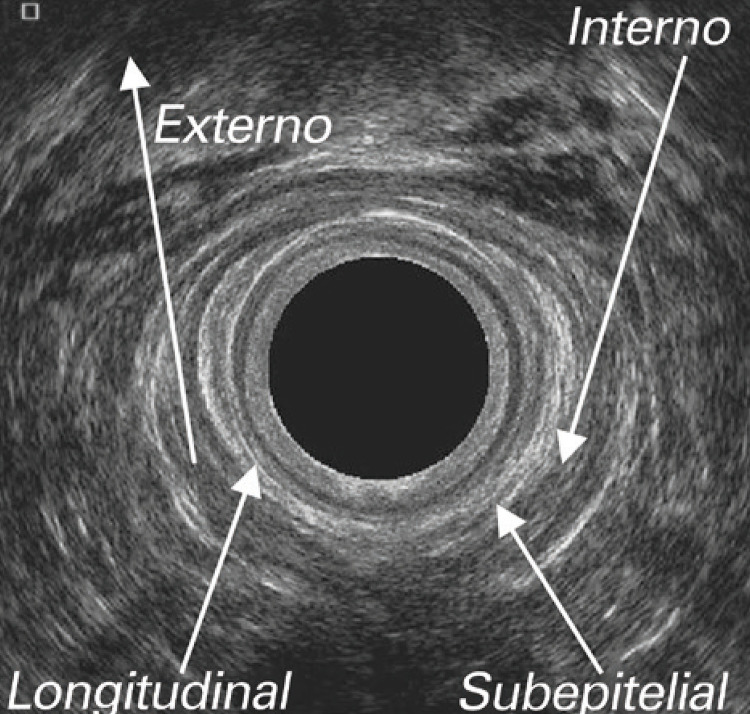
Anatomia normal do canal anal como vista na endossonografia anal4.3 Radiografia4.3.1 Modalidades atuais em uso clínico rotineiro4.3.1.1 Urografia intravenosa: fornece descrição anatômica do trato urinário superior, dos ureteres e da bexiga, bem como a avaliação da função renal e da excreção, do meio de contraste. Urografia intravenosa consiste em pelo menos três a quatro imagens abdominais: um raios X simples, um quase imediatamente após a injeção de avaliar para captação vascular renal, uma imagem aos 7 minutos e outra aos 15 minutos após a infusão de meio de contraste (e esvaziamento de bexiga). A radiografia simples preliminar pode mostrar calcificação no rim, ureter, bexiga, vesículas seminais ou vasos. (
